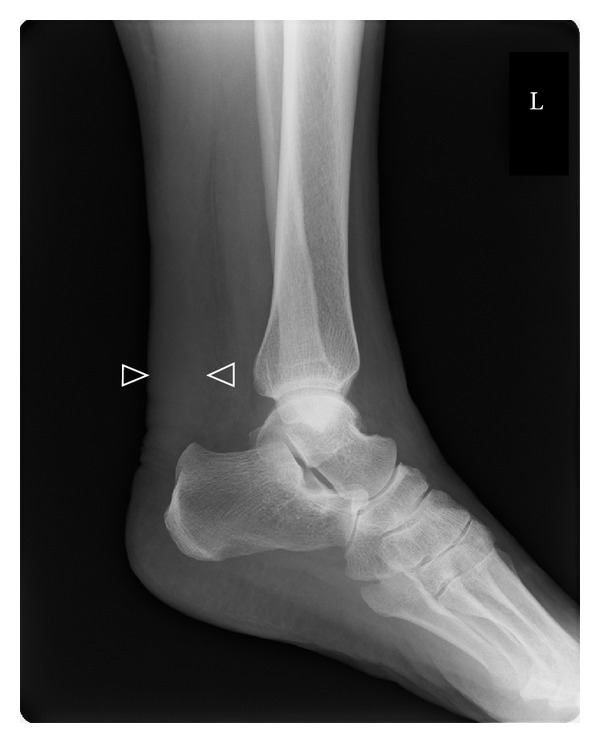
X-ray of the ankle. X-ray of the ankles showed thickening of the bilateral Achilles tendons [left side (arrows heads): 20 mm, right side (not shown): 19 mm].
